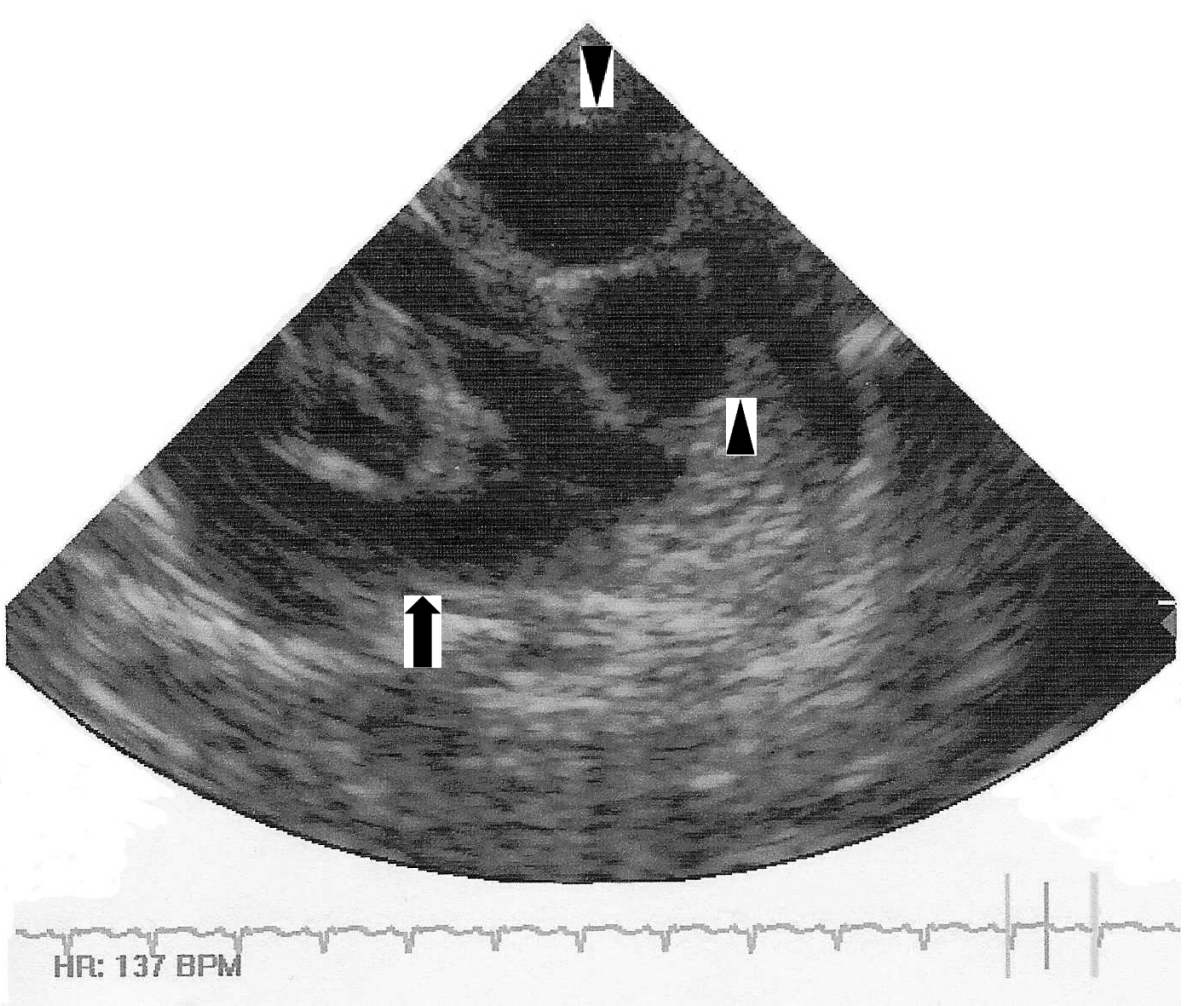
Post-natal echocardiogram showing a multiloculated, cystic mass (arrowheads) separately from the heart (arrow).
